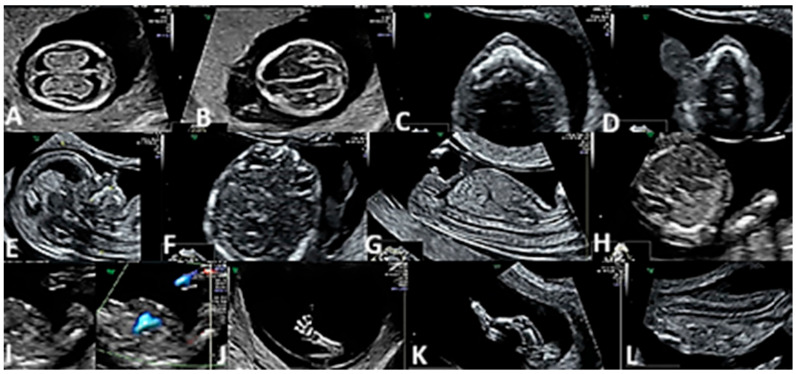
FT ultrasound morphological protocol. (A) Fetal brain (choroid plexus). (B) Fetal brain (thalamus and median septum). (C) Fetal face (upper lip and anterior palate). (D) Fetal face (lower lip and mandible). (E) Face sagittal view. (F) Fetal face (orbits and lens). (G) Fetal thorax and abdomen. (H) Fetal heart (4CV). (I) Fetal heart (3VV in Gray Scale and Color Doppler Mode). (J,K) Fetal limbs (upper and lower limbs). (L) Fetal spine.
